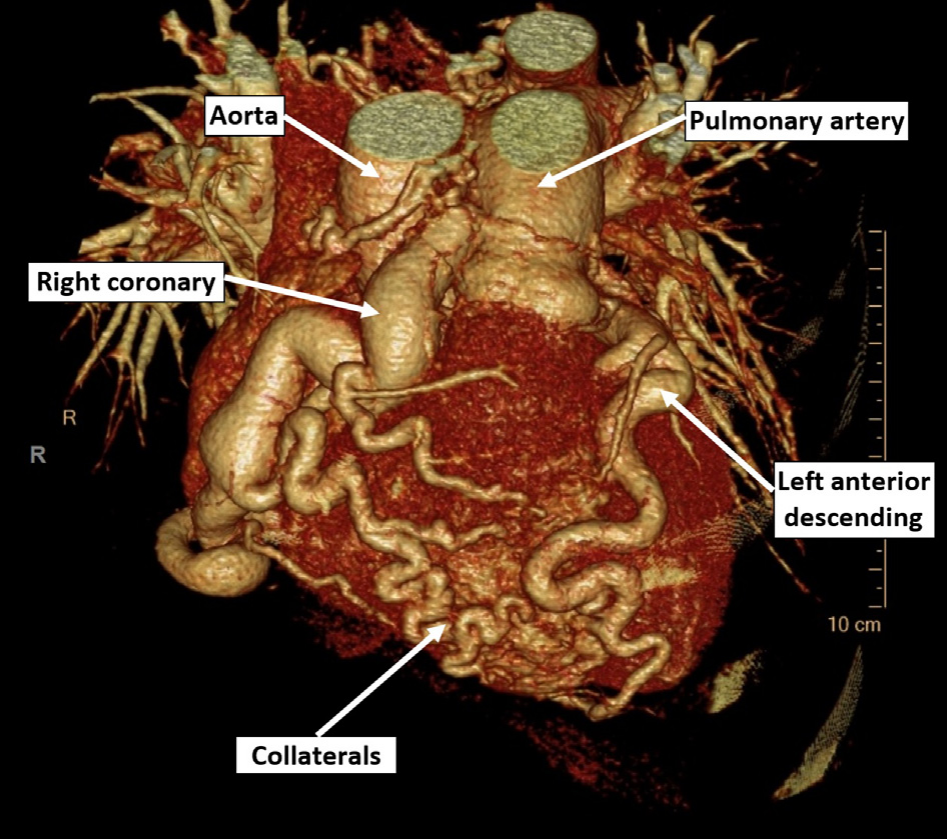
Cardiac CT 3D reconstruction showing dilated LAD and the anomalous dilated and aneurysmal RCA that arises from the pulmonary artery.
